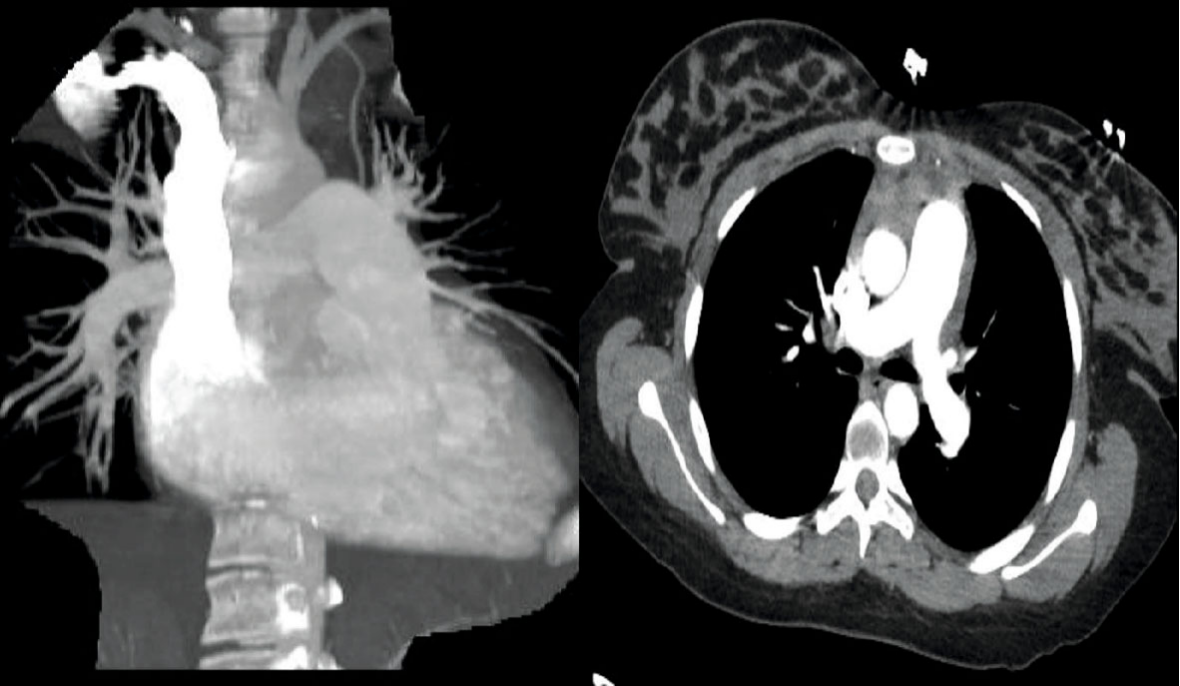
CT pulmonary angiogram showing dilated pulmonary trunk, with no evidence of pulmonary embolism.
